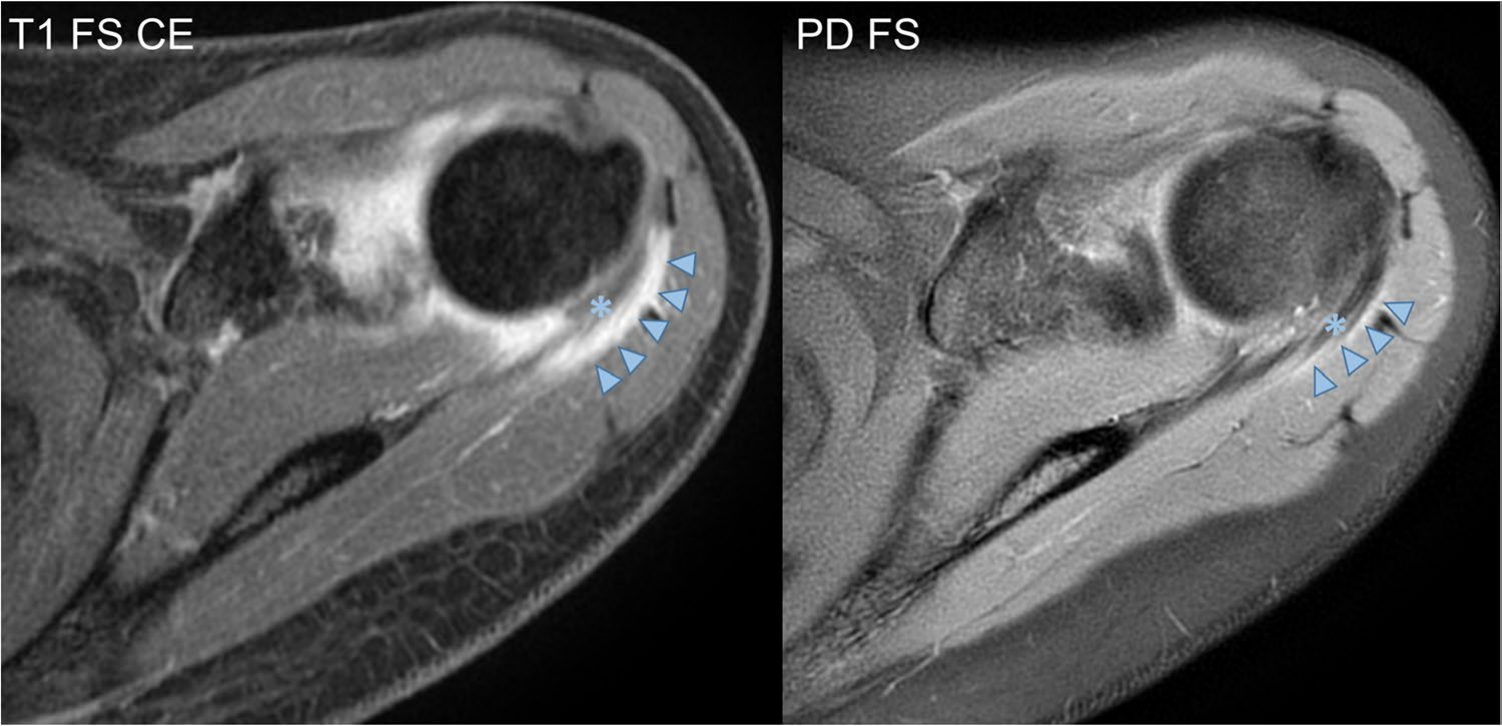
Infraspinatus tendonitis in a 22-year-old female—on the T1-weighted fat-saturated contrast-enhanced (CE) image, avid enhancement is evident (arrowheads) surrounding the M. infraspinatus tendon (*); by comparison, subtle oedema is present surrounding the tendon on the fluid-sensitive, fat-suppressed image (PD FS)
